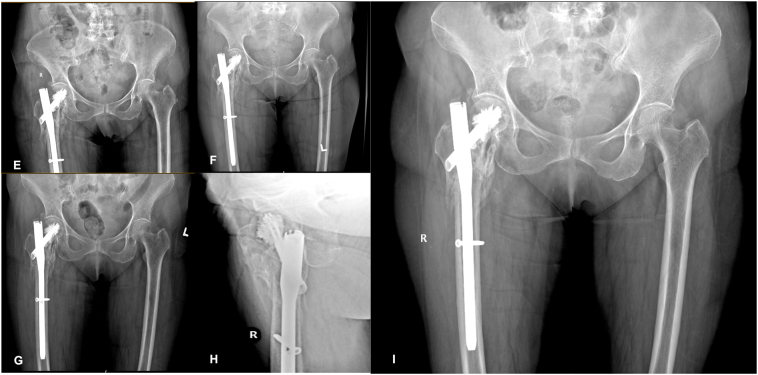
A–D): A) Pre op radiograph AP view and B) lateral view showing IT fracture. C: Post-operative radiograph at 6 weeks AP view and D) lateral view of TFNA fixation of fracture. E–I: E) Post-operative radiograph at 16 weeks, F) at 7 months follow up, G&H) Ap and lateral view radiograph at 11 months follow up and I) AP view at 15 months follow up.
